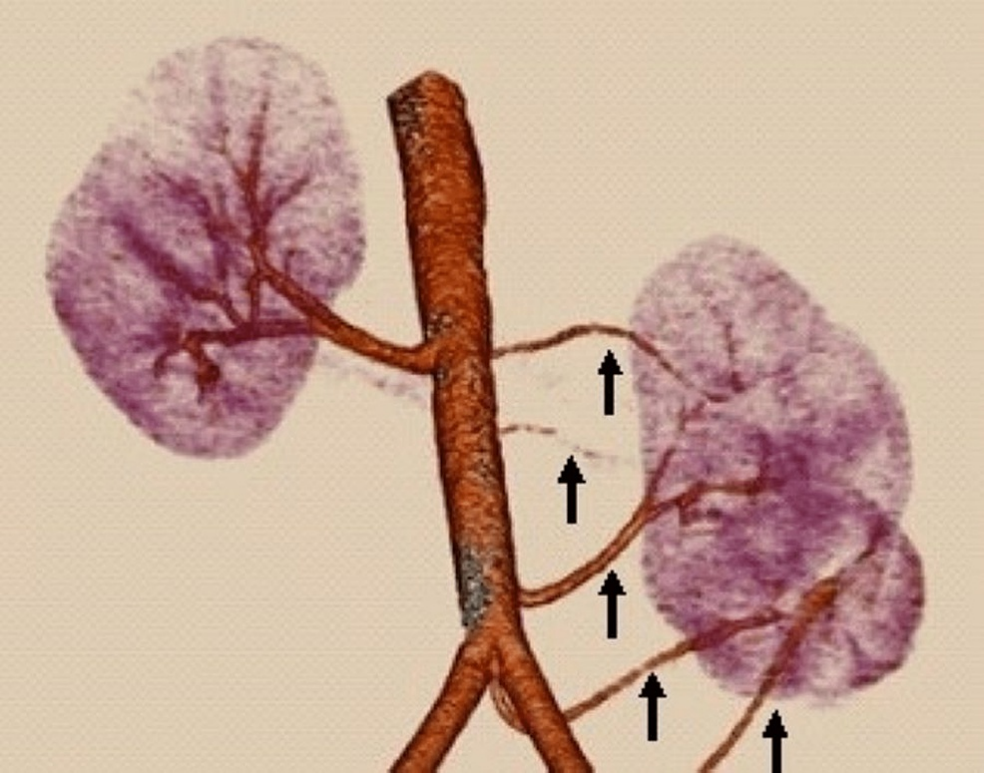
Multiplanar reconstruction image of CT angiography demonstrates five left renal arteries with separate aortic origins.
